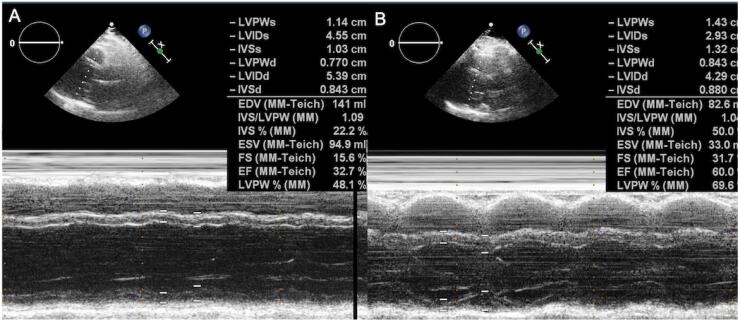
Changes in the pre-treatment (A) and post-treatment (B) left ventricle function in M-mode echocardiography.
